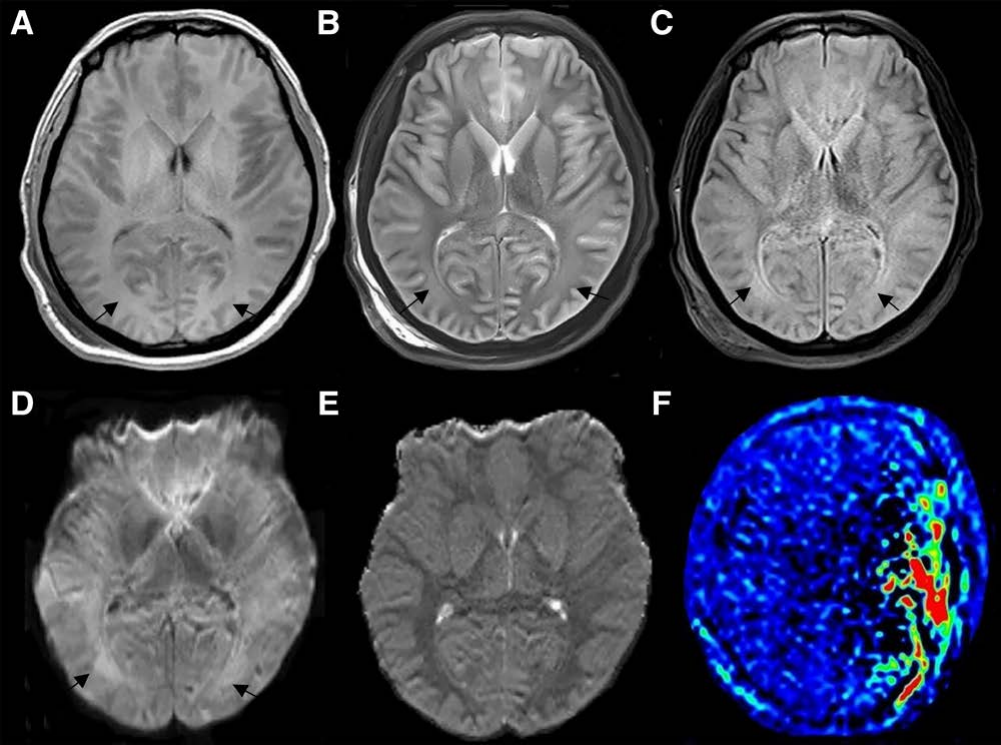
MRI of the head. Symmetrical distribution of low-signal lesions in T1 sequences (A). Low-signal lesions in T2 sequences (B). And a high FLAIR sequence signal (C). Restricted symmetric diffusion in both cerebral hemispheres (D, E). And asymmetrical reduced perfusion of brain tissue (F). The black arrow demonstrates symmetric involvement of the brain parenchyma. FLAIR = fluid-attenuated inversion recovery, MRI = magnetic resonance imaging
